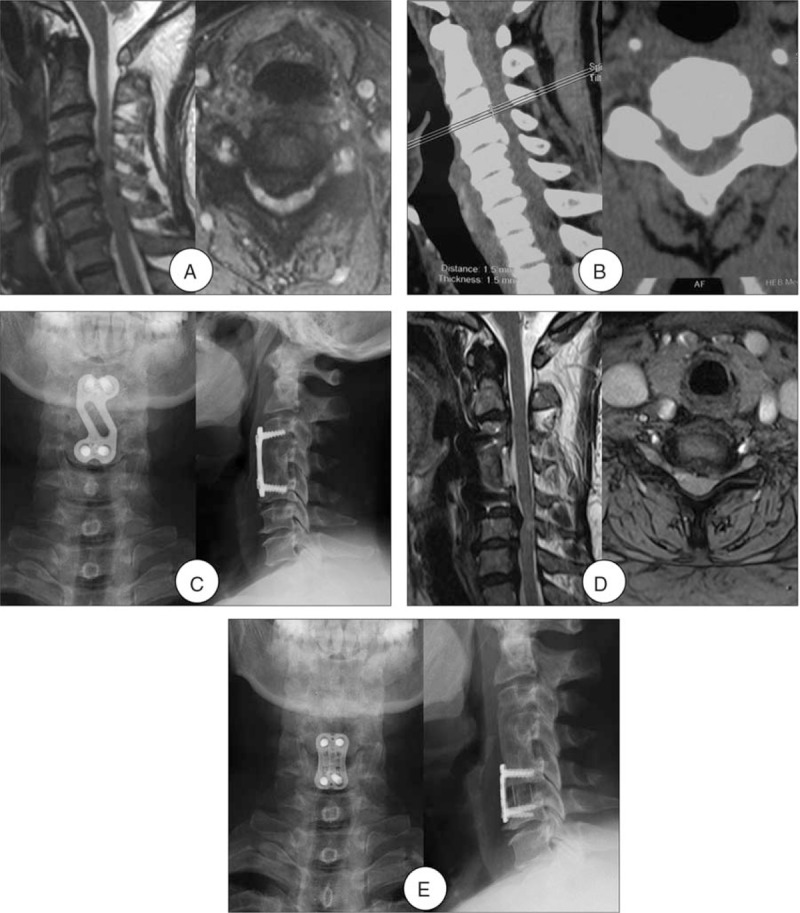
A 52-year-old woman developed one-level ASD 7 years after initial surgery. (A) Preoperative MRI of this patient shown severe compressions of the spinal cord at C4-5 level as well as high signal intensity in the spinal cord. (B) Preoperative CT shown ossification formation at C4-5. (C) Radiograph after initial surgery shown ACCF at C4. (D) MRI at 7-year follow-up shown development of ASD and spinal cord compression at C5–6. (E) Radiograph after the reoperation shown ACDF at C5-6 and the fixed plate of the initial surgery was removed. ACCF = anterior cervical corpectomy and fusion, ACDF = anterior cervical decompression and fusion, ASD = adjacent segment disease, CT = computed tomography, MRI = magnetic resonance imaging.
